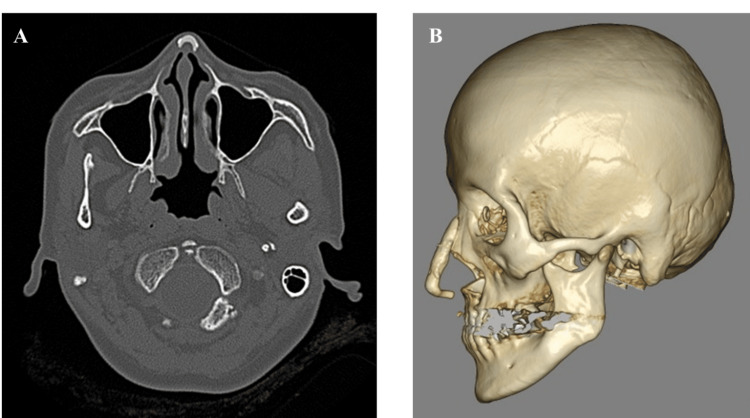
Preoperative CT scan images (A) Coronal section and (B) 3D reconstruction revealing an L-shaped silicone implant extending from the nasal dorsum to the base of the columella
